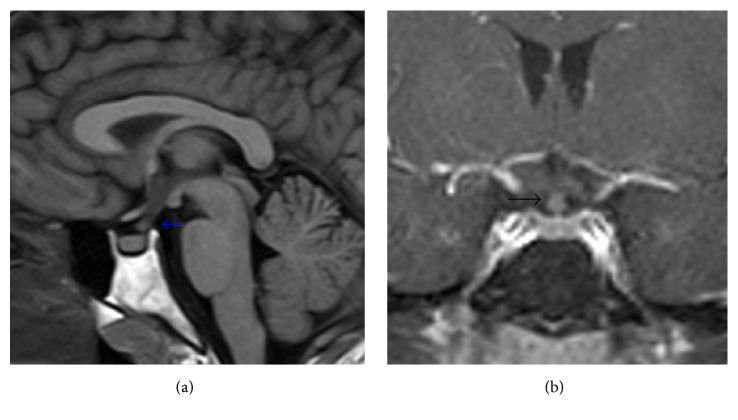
(a) Sagittal T1-weighted MRI of the sella turcica showing a thick pituitary stalk (arrow). Please note the absence of expected hyperintense (bright) signal of the neurohypophysis. (b) Coronal T1-weighted post-Gd MRI showing a thick pituitary stalk (arrow).
